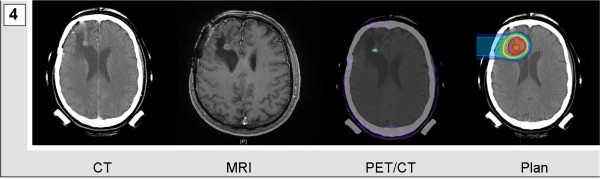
Nodular atypical meningioma in a 50-year-old man. Contrast-agent enhanced CT and MRI scan were fused with a DOTATOC-PET/CT examination and used to calculate a single beam carbon ion radiotherapy plan.
